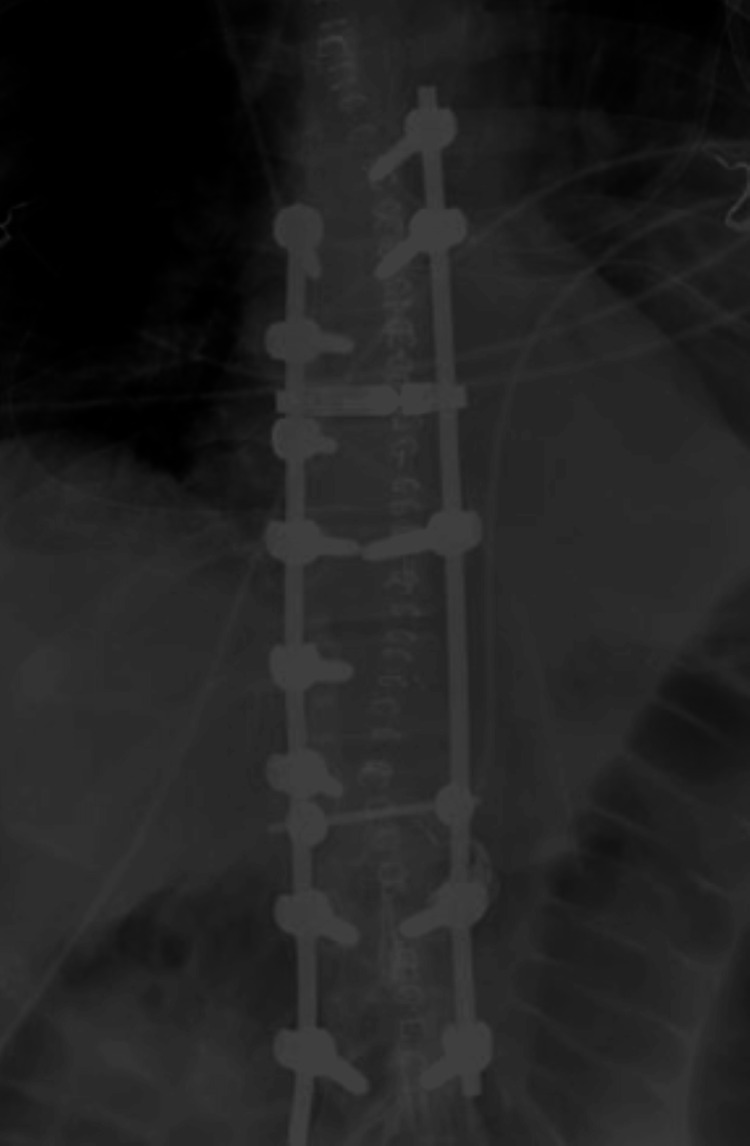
Post-operative X-ray Immediate post-operative coronal X-ray showing posterior instrumentation
